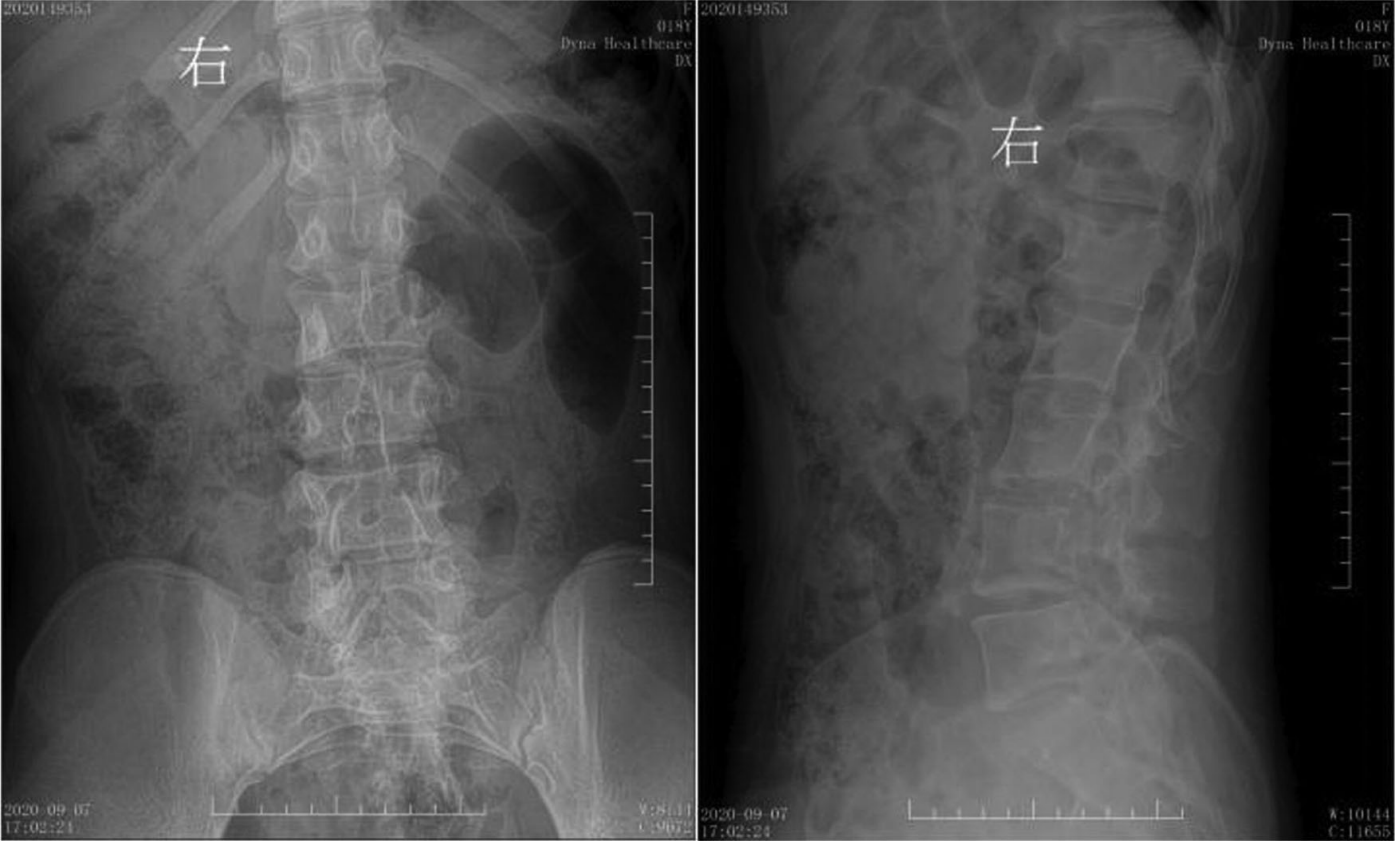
Preoperative digital radiography. Spina bifida is shown in the lumbar spine, with the L3 spinous process extending into the spinal canal.
